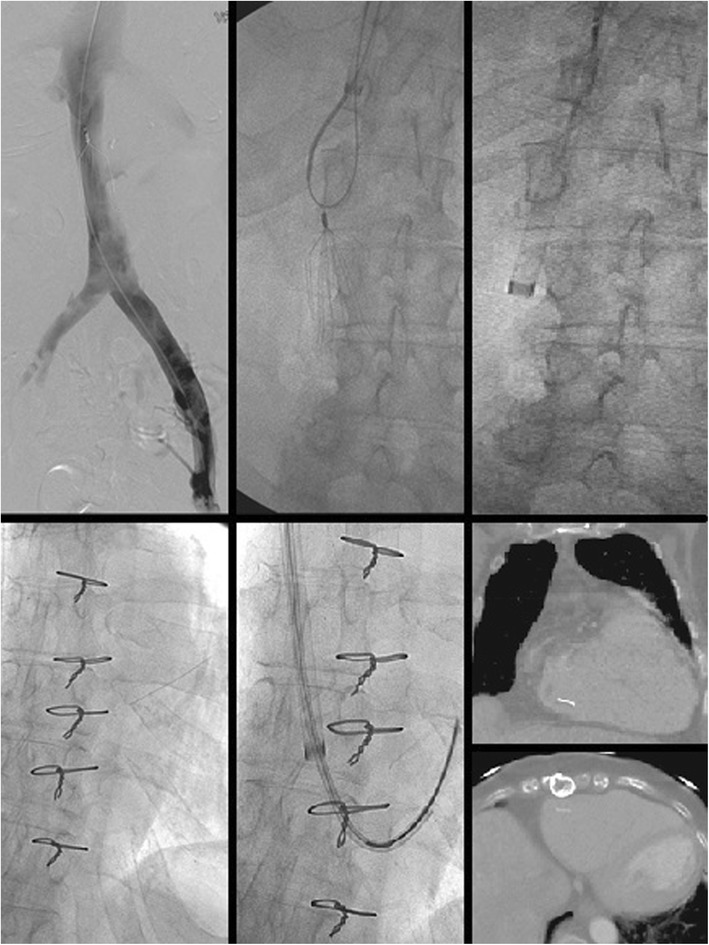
Initial cavagram shows an intact Bard Eclipse filter (Bard Peripheral Vascular, Inc., Tempe, AZ) IVC filter. Simple loop snare was unsucessful because of posterior tilt and embedded hook. The Hangman’s technique, a modification of the sling technique where a glidewire loop is created using a reversed curved catheter is created between the caval wall and filter hook to separate the tip of the filter from the wall of the filter, was used. After sucessful filter removal, ex-vivo examination showed two missing filter legs. Subsequent images showed one piece to be in the pulmonary outflow tract and one in the right atrium. The larger fragment was sucessfully retrieved from the pulmonary outflow tract but the piece in the right atrium could not be removed. Subsequent echocardiogram showed new severe tricuspid regurgitation which was managed medically. In situ leg fracture of certain types of filters is higher than others. The older generation Bard filters have a 25% rate of in situ leg fracture (Nicholson et al. 2010). While no published data exists on differential rates of fracture during retrieval by filter type, it is likely that filters prone to spontaneous in situ fracture are also more likely to fracture when subjected to mechanical stresses during retrieval
